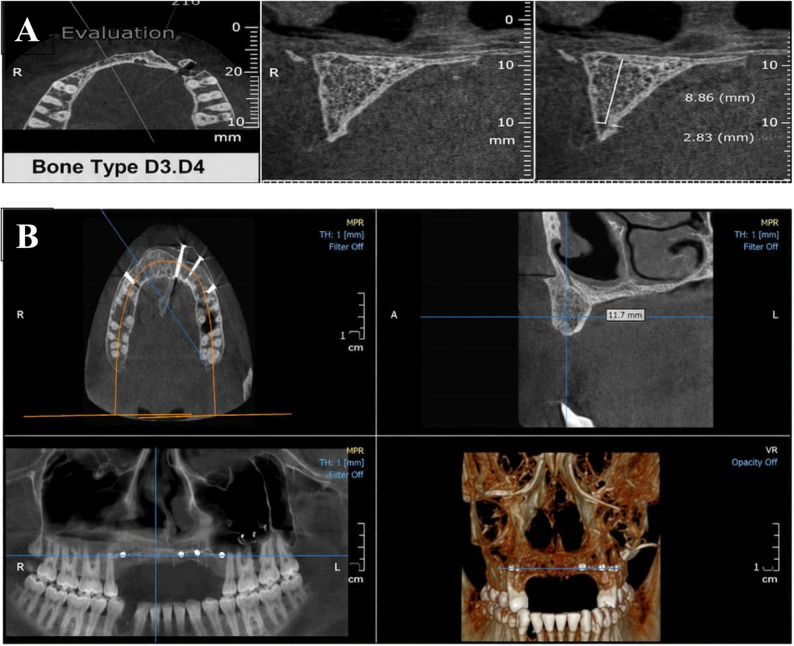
CBCT radiographic assessment of alveolar ridge augmentation using iliac crest bone grafting. A Preoperative CBCT images demonstrating traumatic alveolar ridge deficiency with reduced ridge width and inadequate bone volume for implant placement. B Postoperative CBCT images after graft consolidation showing increased alveolar ridge thickness and improved bone volume prior to delayed implant placement
